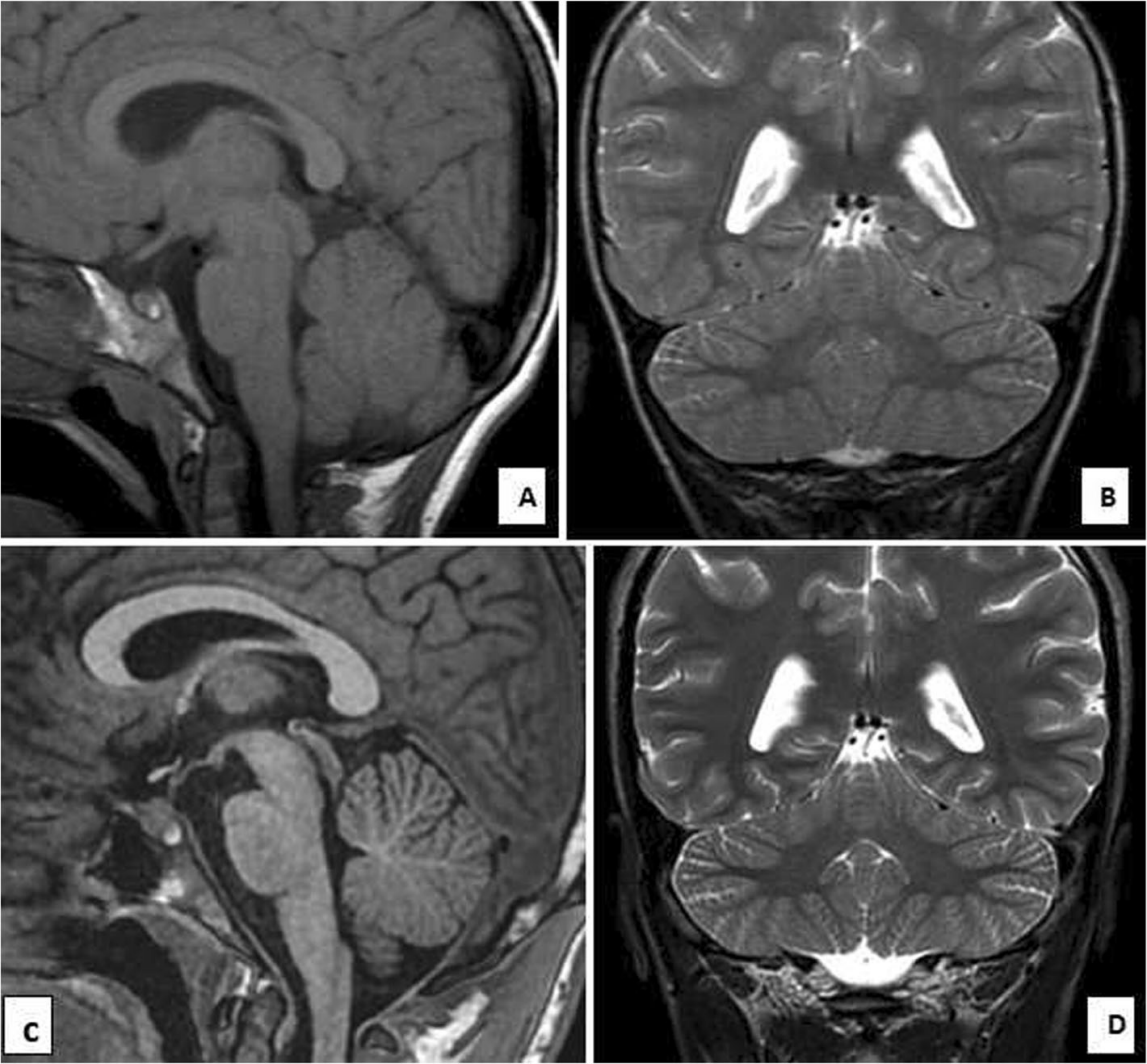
Patient 2. a and b MR images at the age of 6 years (a) Sagittal T1 and (b) Coronal T2- weighted MR images of the cerebellum showing normal cerebellar volume and folia with normal vermis and no signs of atrophy. c and d MR images at the age of 16 years (c) Sagittal T1 and (d) Coronal T2-weighted MR images of the cerebellum showing minimal superior vermian atrophy. The other parts of the cerebellum show normal volume and folia with no signs of atrophy. The cerebellar gray and white matter are also normal
